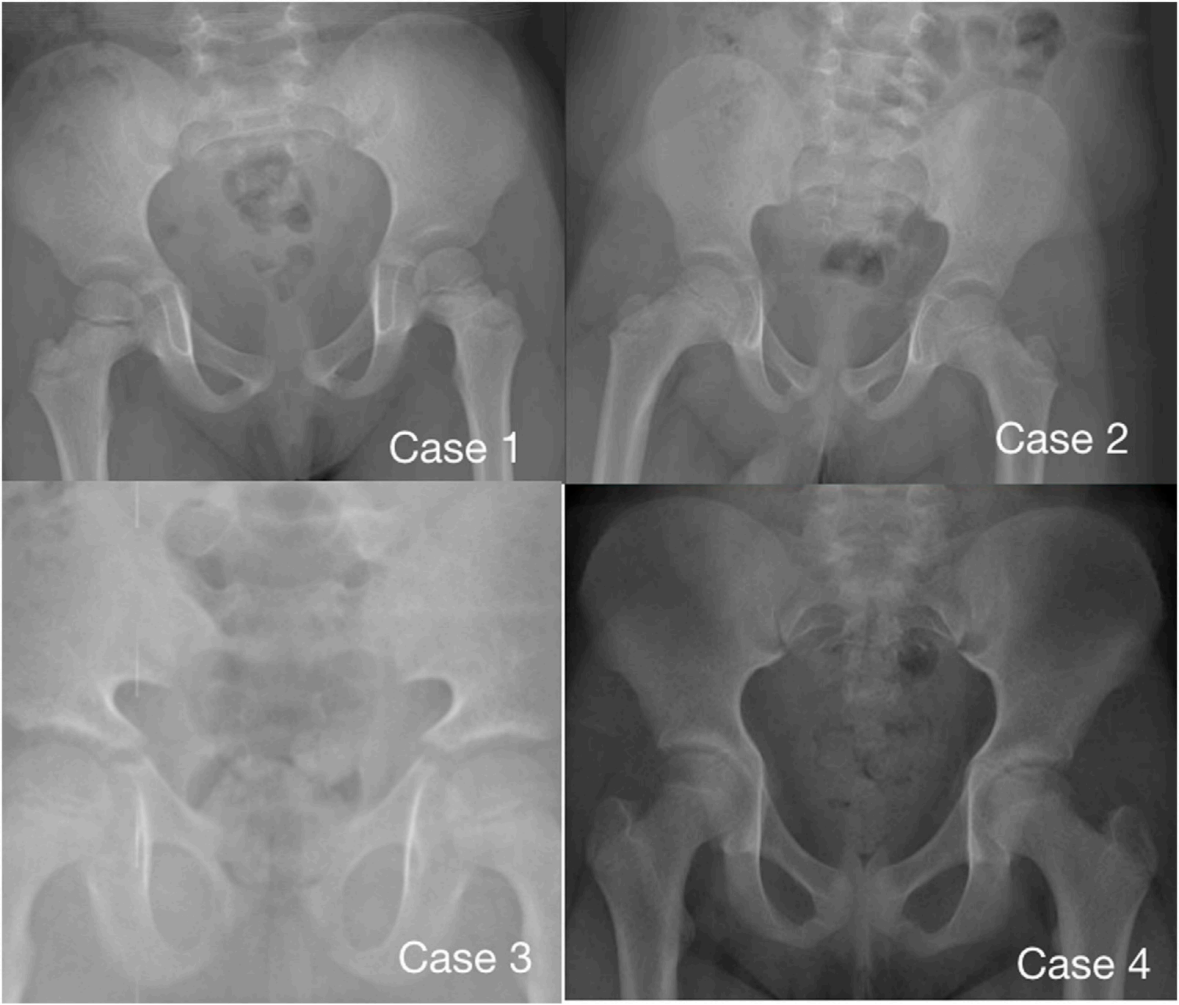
Pelvic radiographs of 4 children with 3M syndrome. No obvious abnormality was found in Case 1. The pelvic measurements of Cases 2, 3 and 4 are smaller than those of their peers. Case 3 shows a typical triangular pelvis.
